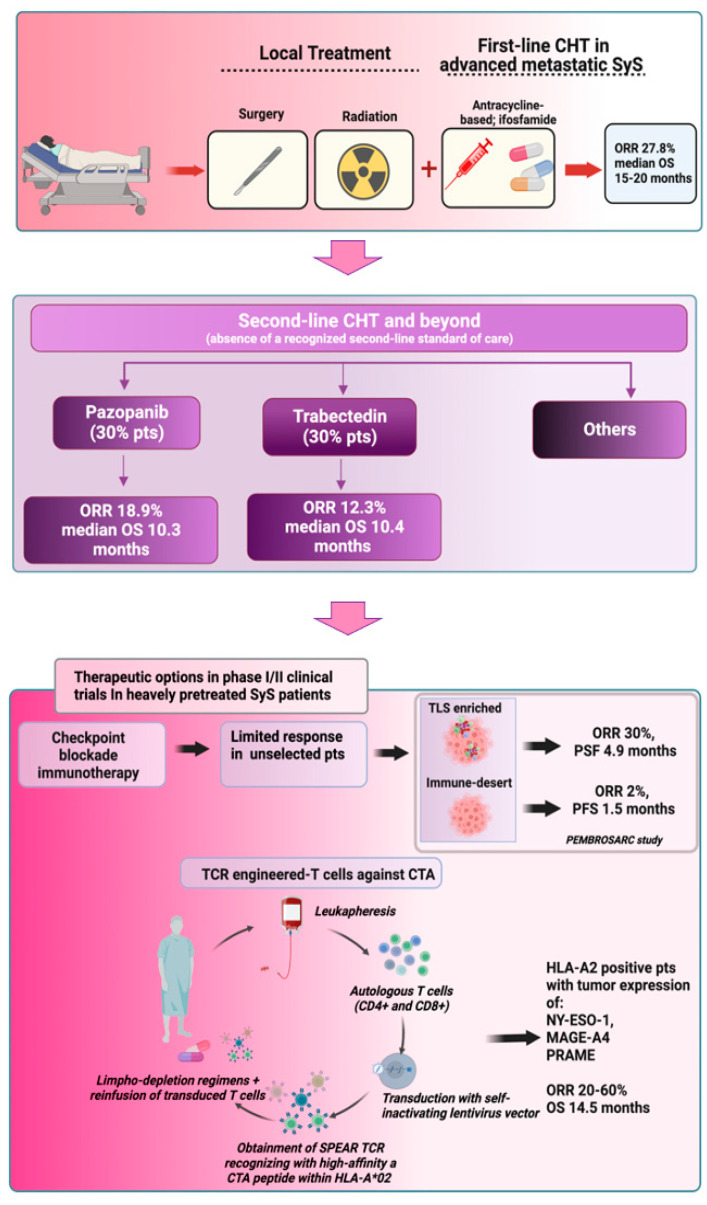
Current therapeutic options for advanced, unresectable, metastatic SyS. After local therapies and front line anthracycline and ifosfamide-based chemotherapy (CHT) [4], patients showing disease relapse undergo second line CHT with decreased ORR and OS [25,30,31]. Immunological therapies under evaluation in phase I/II clinical trials show limited results for ICI in SyS patients, but improved outcomes can derive from the use of tumor immune microenvironment biomarkers for the selection of patients more likely to respond to ICI-based therapies [50,51,62,63]. Higher ORR can be observed in HLA-A2 positive patients receiving TCR-engineered T-cells recognizing cancer/testis antigen (CTA) peptides [66,68,69,70,74,75,76,79] (created with Biorender.com accessed on 25 July 2023).
